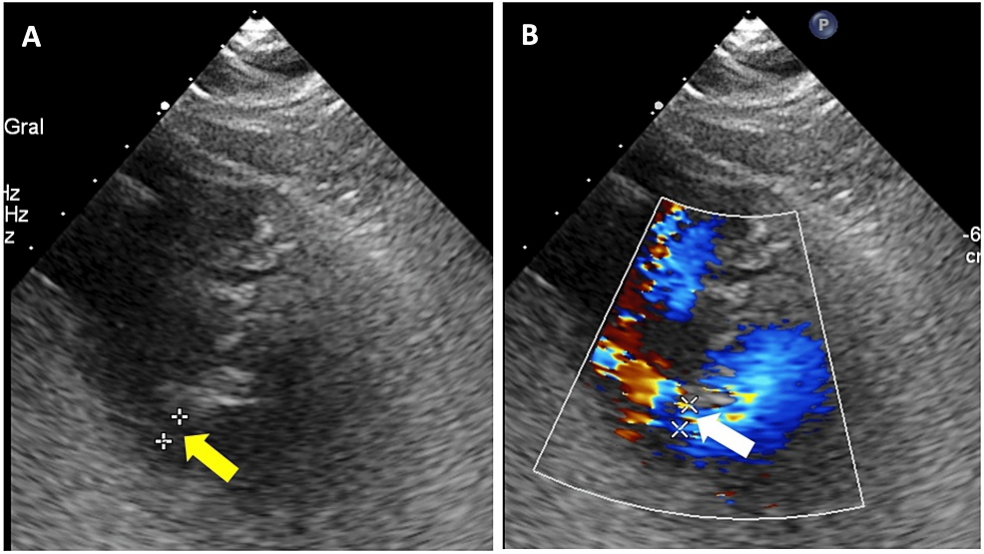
Pre-discharge transthoracic echocardiography. (A) Parasternal short-axis view showing a residual ventricular septal rupture (VSR) of 3 mm (yellow arrow). (B) Color Doppler in the area of the residual VSR (white arrow).
